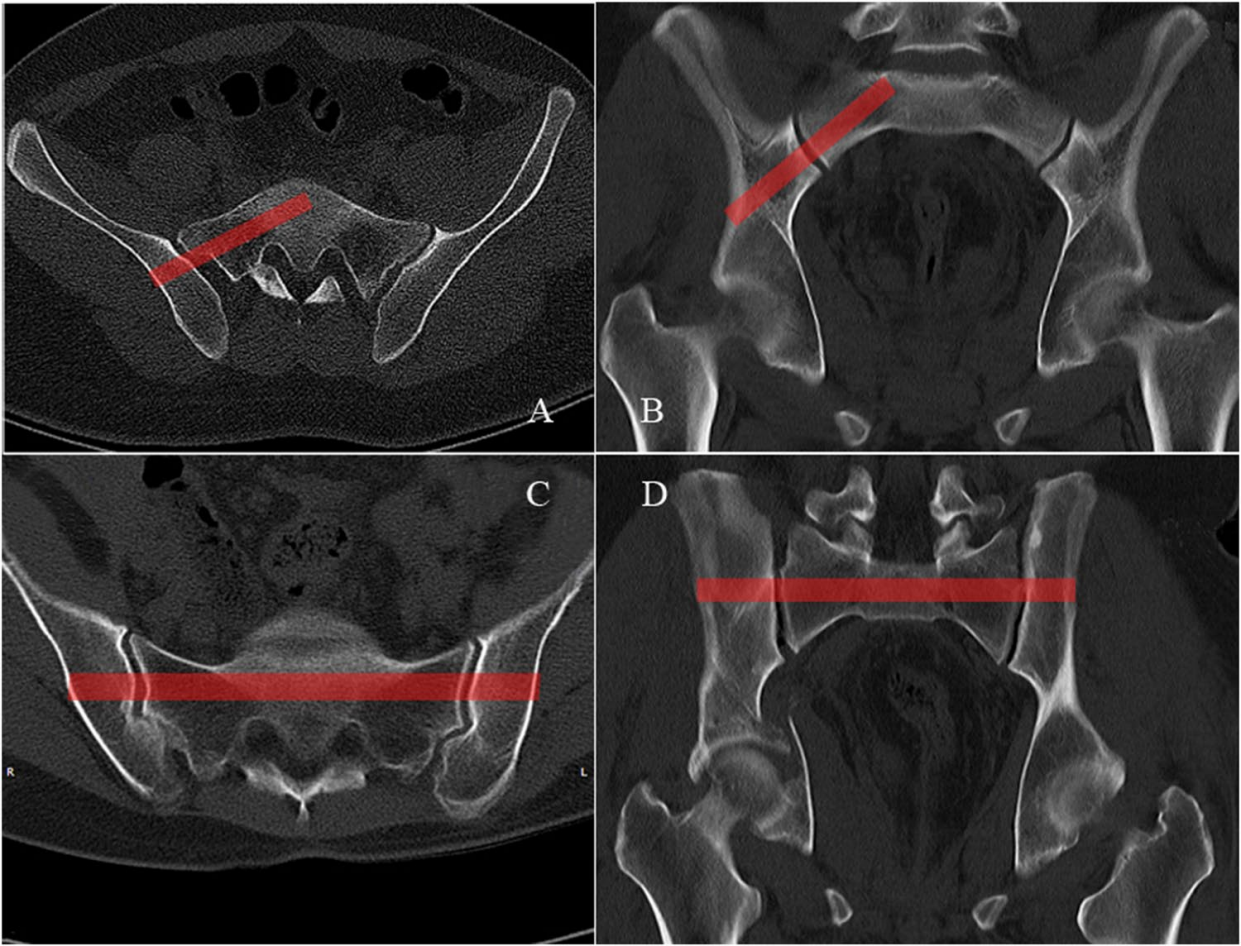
(A,B) The top sacral segment axial CT imagery of a patient with sacral dysmorphism. The dysmorphic sacrum has an oblique osseous pathway, which only allows oblique screw placement. (C,D) The top sacral segment axial CT imagery of a patient with a normal sacrum. The red column represents the pathway available for transverse screw placement.
